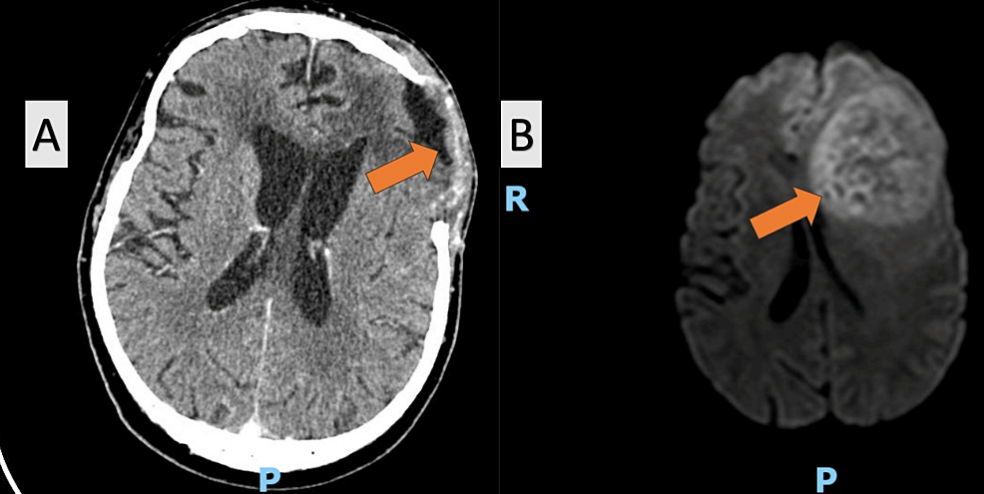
(A) Postoperative CT scan, (B) preoperative MRI scan
